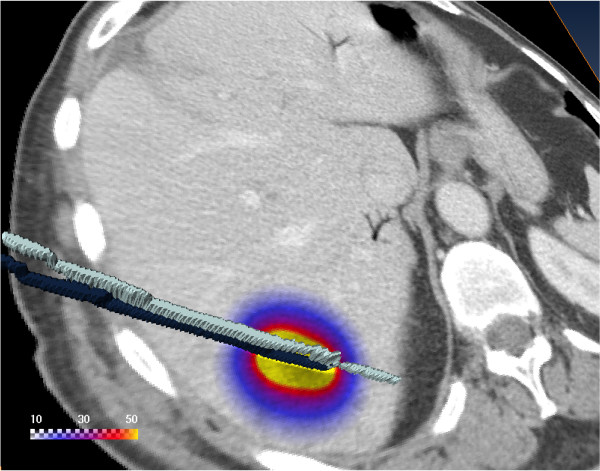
Geometry. The 3D visualization shows a CT slice with the calculated dose in Gy overlayed. The dose is applied using two catheters. The two catheters were visualized in 3D using surface rendering of the catheters labeled in the CT scan.
